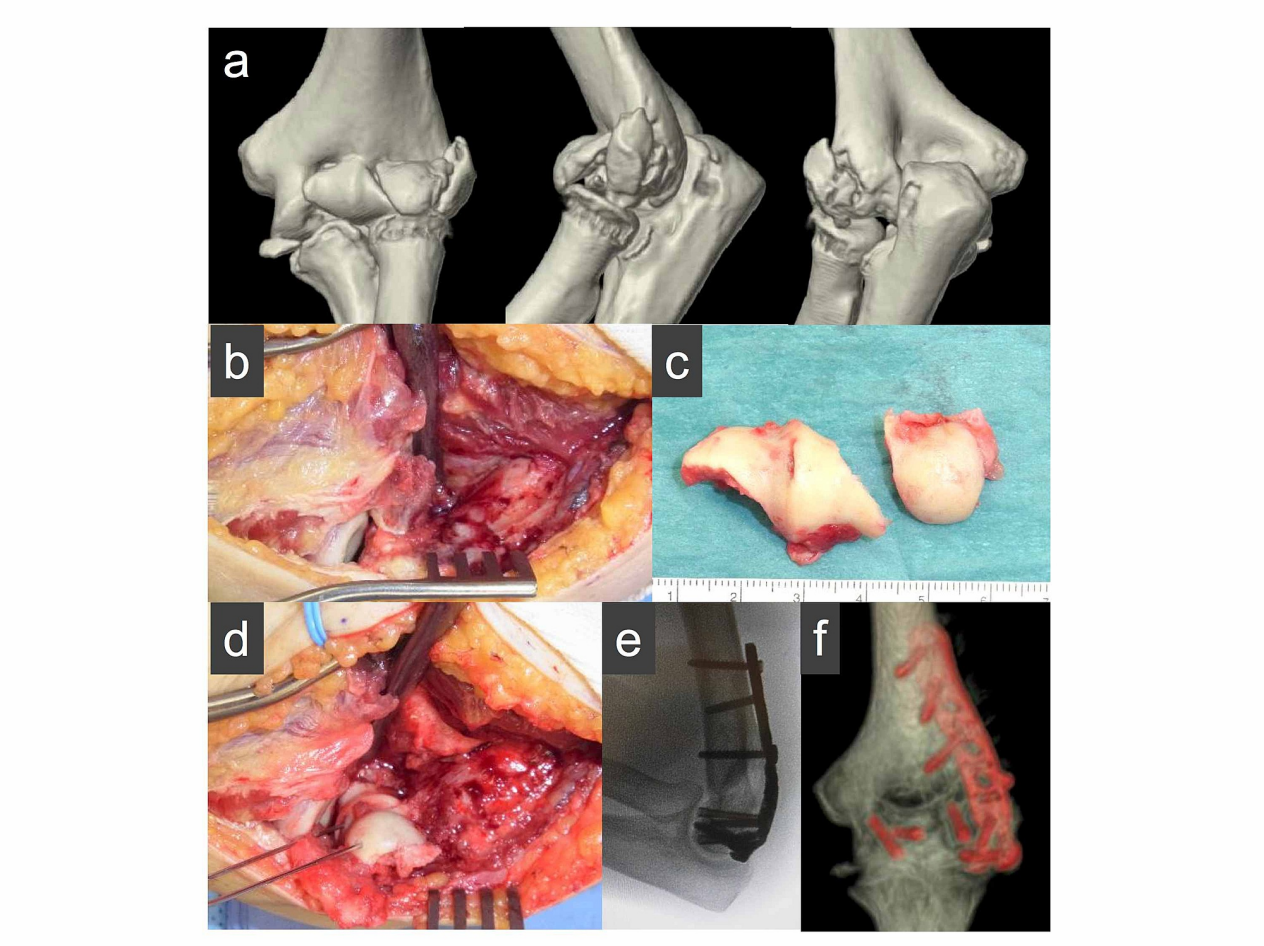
(a) Preoperative CT scan of a 72-year-old female patient with a Dubberley type 2B capitellum fracture with posterior comminution. An extensile Kocher approach was utilized, and the capitellum and trochlea fragments were removed (b, c) and re-affixed with provisional K-wires (d). (e) Postoperative X-ray shows adequate congruency of the radiocapitellar joint and (f) CT shows stable fixation with a posterior distal humerus locking plate.
